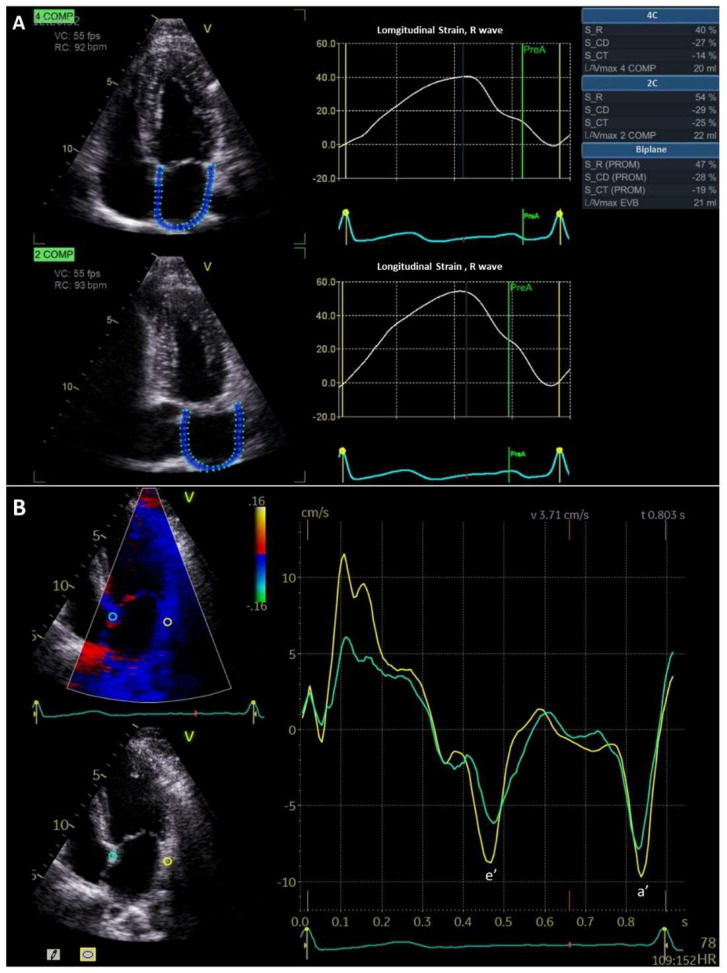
Atrial function evaluated by speckle tracking (A) and tissue Doppler imaging (B). Left atrial strain curves (A) with R wave as time reference, showing the 3 phases of the atrial function measured in apical four and two chamber views: reservoir longitudinal strain (47%) is the peak positive value, conduit longitudinal strain (−28%) is the measurement during early diastole and contractile longitudinal strain (−19%) is the value during end-diastole. Tissue Doppler imaging in the apical four chamber view to assess regional LA function (B). The samples are placed in atrial (green) and in the lateral wall (yellow). Peak diastolic velocities are assessed. Early diastolic filling is indicated by e’ and end-diastolic filling with a’.
